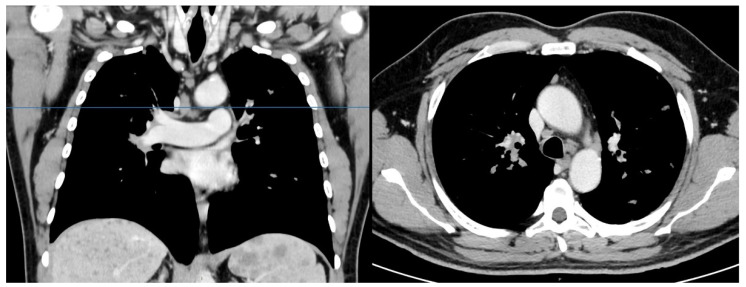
Chest computed tomography showed some slightly enlarged mediastinal lymph nodes.
